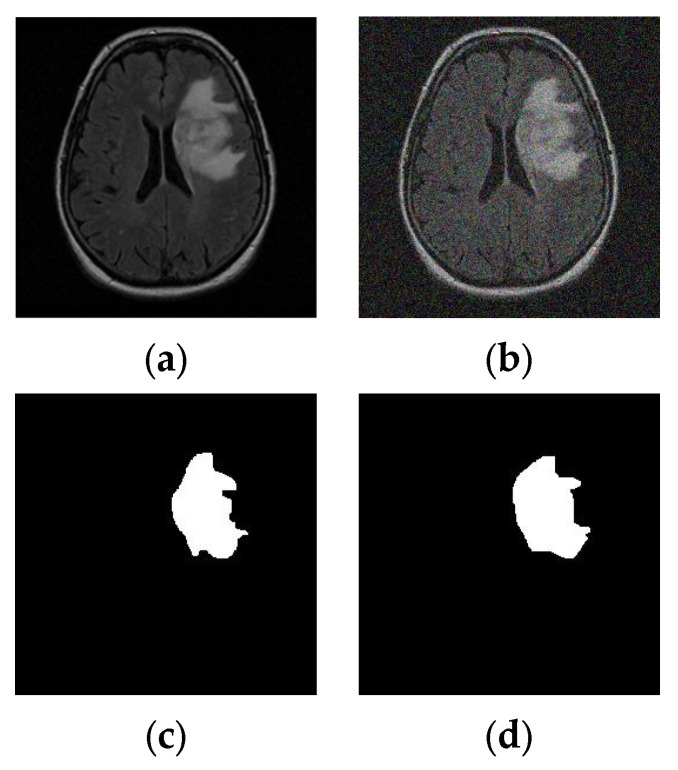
Tumor-section extraction from the chosen MRI slice with the watershed algorithm. (a) Original image, (b) noisy image, (c) tumor from original image, (d) tumor from noisy image.
